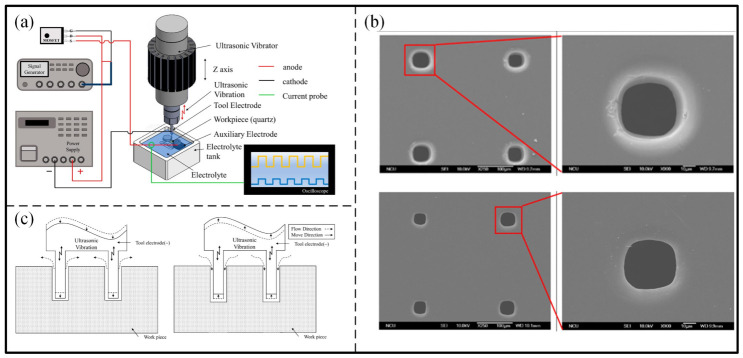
Ultrasonic vibration-assisted ECDM of quartz wafer micro-through-hole arrays [41]: (a) experimental setup; (b) inlet and outlet morphologies at 44 V; (c) electrolyte pumping effect induced by ultrasonic vibration.
